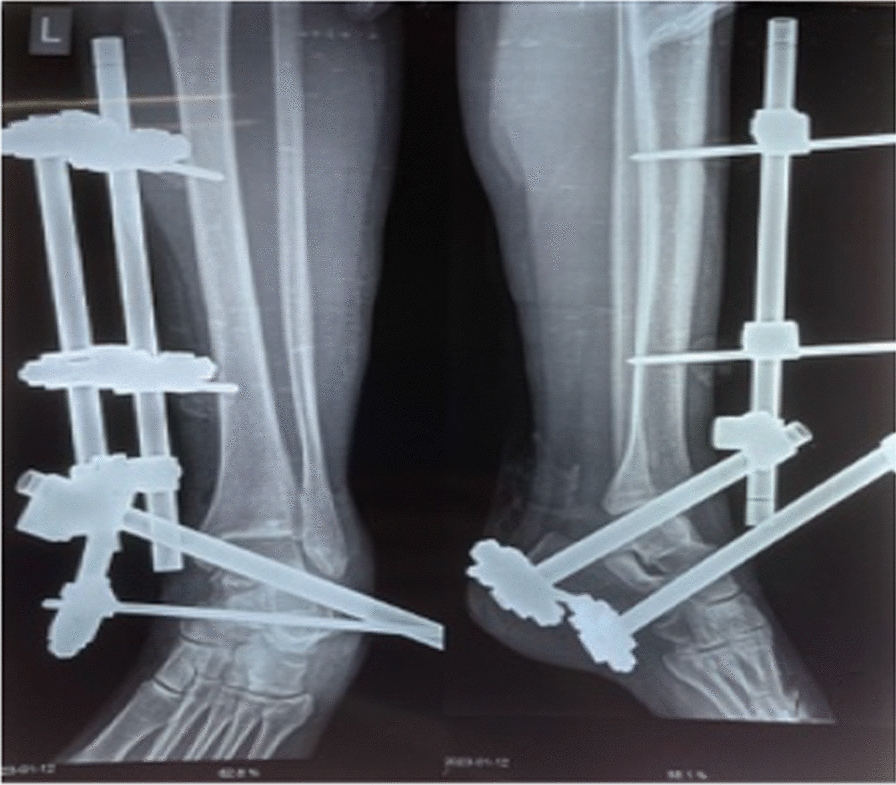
Radiograph post operation showing reduction of talus with external fixator in place
